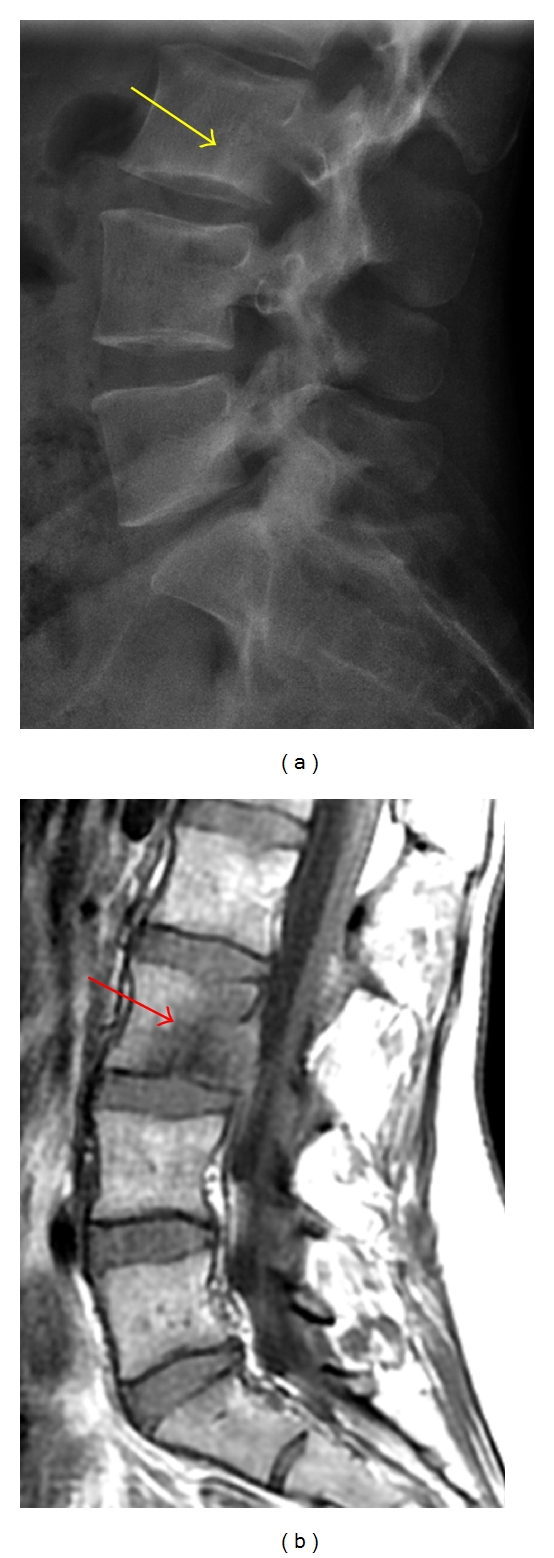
(a) Lateral radiograph is poor at delineating the L3 vertebral body metastatic lesion, which appears as a faint lucency with a subtle sclerotic margin (yellow arrow). (b) This lesion is better seen on the sagittal T1-weighted MRI as an ill-defined hypointensity (red arrow) within the L3 marrow.
